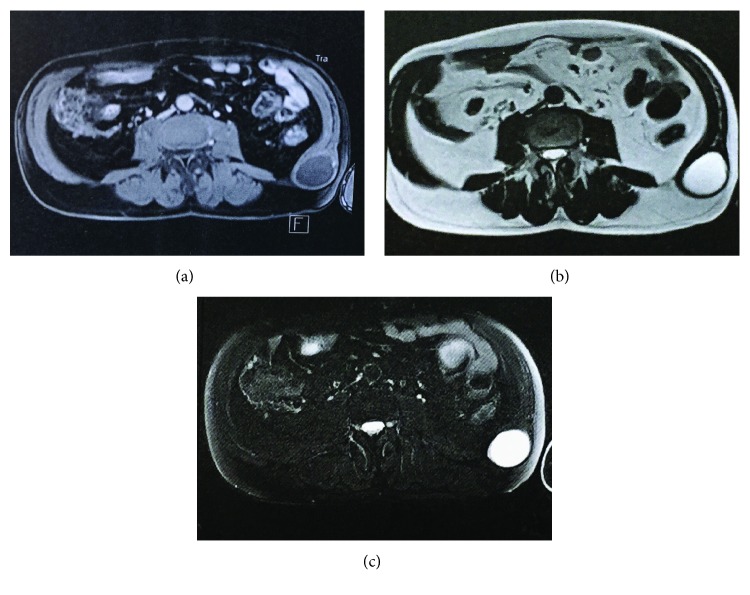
(a) Transverse section of postcontrast T1 MRI abdomen revealed a well-circumscribed mass located between the external and internal oblique muscles. (b) T2W MRI clearly delineates the capsule of the lesion and internal morphology. (c) The lesion is intensely hyperintense on fat-suppressed MRI.
